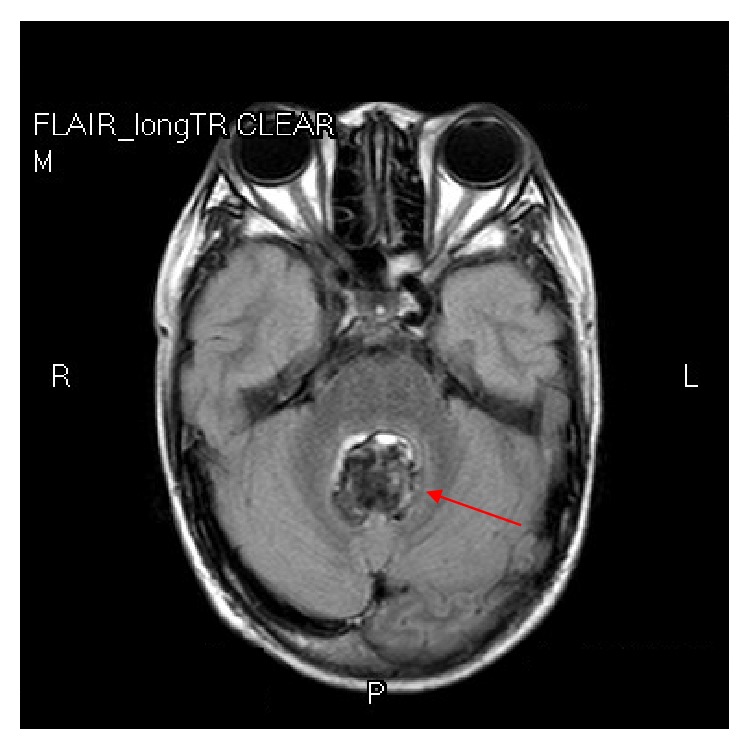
Magnetic resonance imaging (FLAIR, axial view) showing a lobulated, well-defined intraventricular mass (arrow) with no paraventricular extension attaching the fourth ventricular floor.
